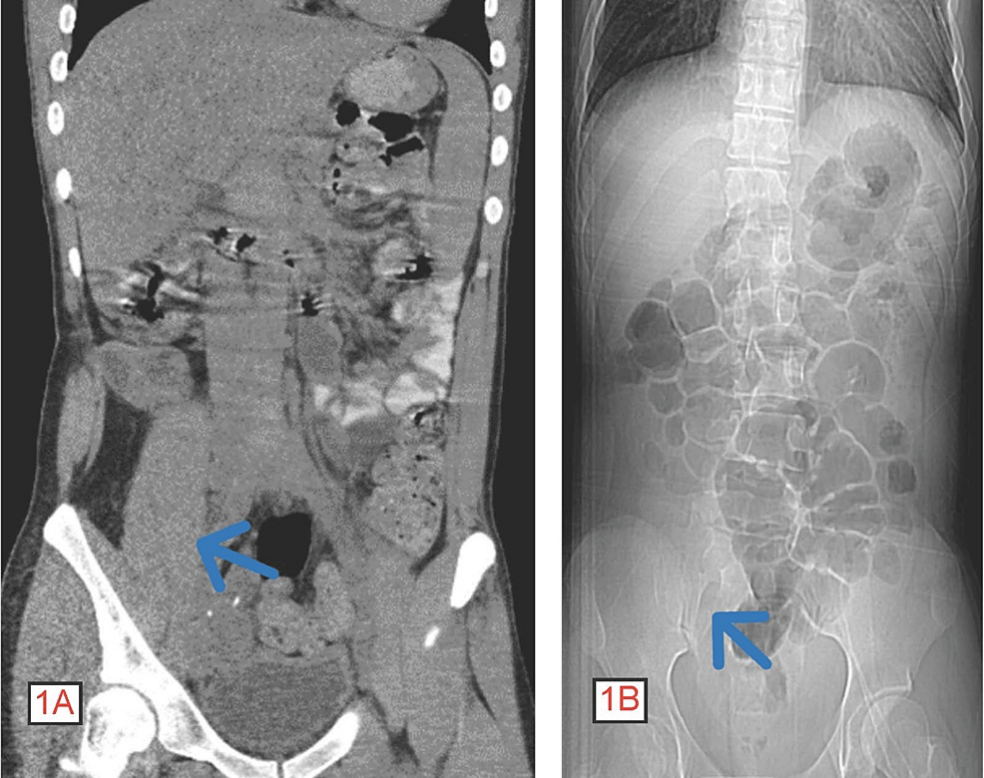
Computed tomography (CT) of the abdomen without contrast depicting no bowel obstruction, an unidentifiable appendix, and no inflammatory changes in the right lower quadrant
